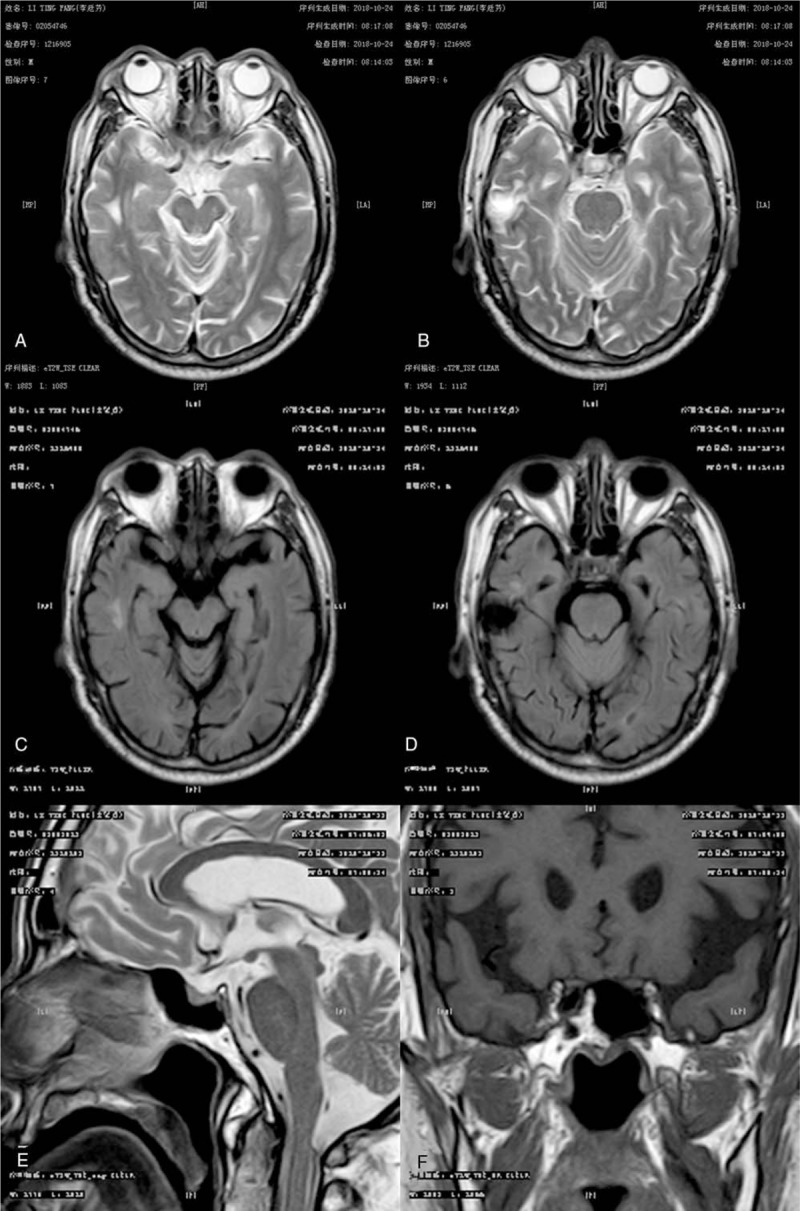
Brain magnetic resonance imaging. Brain magnetic resonance imaging showed no abnormality (A and B: axial T2-weighted imaging; C and D: axial T1-weighted imaging; E: sagittal T2-weighted imaging; F: coronal T1-weighted imaging).
